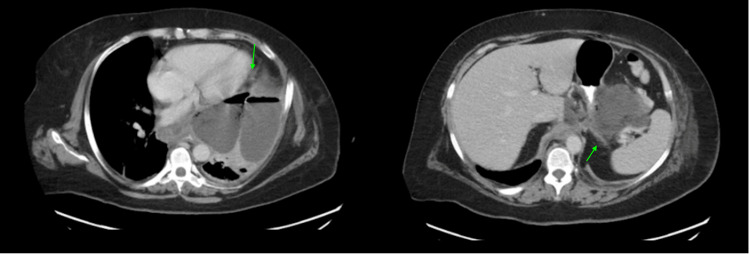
Repeat CT C/A/P revealed a mild interval increase in the peripancreatic fluid collection size concerning for persistent leak, increasing mass effect on cardiomediastinal structures, and loculated thoracoabdominal fluid collections.
